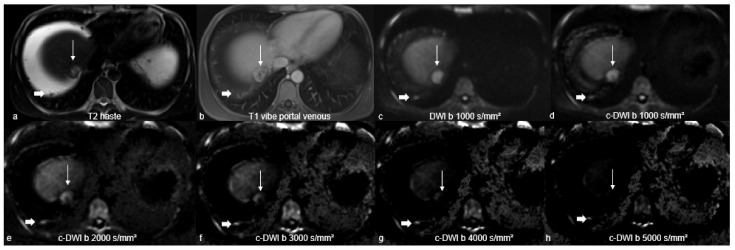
A representative patient of the patient sample with peritoneal carcinomatosis with primary cancer of hepatocellular carcinoma located perihepatically (thin arrow) on T2-weighted sequence (a), fat-saturated T1-sequence after contrast agent administration (b), axially acquired (c), and computed diffusion-weighted imaging at high b-values of 1000–5000 s/mm² (d–h). On DWI with a b-value of 1000 s/mm² (c), peritoneal carcinomatosis is as well visualised as on c-DWI with b-values of 1000 (d), and is better visualised than on c-DWI images at higher b-values (2000–5000 s/mm²). Starting from high b-value of 2000 s/mm² (e), there is an increasing degradation of image quality. Note the additive discrete peritoneal nodules perihepatic (thick arrow) adherent to the peritoneum with concomitant ascites.
